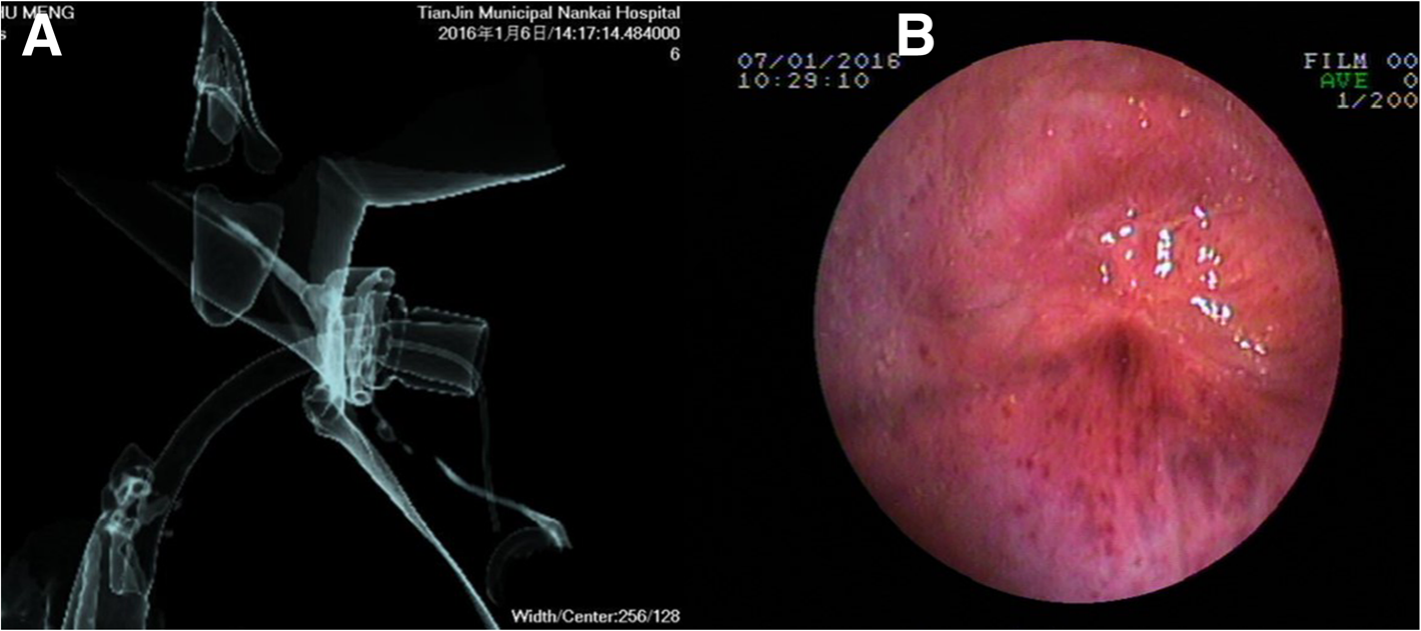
Preoperative examination. a: CT showed that the stenosis was 2 cm long. b: Bronchoscopy showed that the stenosis was at 2.5 cm below the glottis and the surface of the stenosis was completely epithelialized
